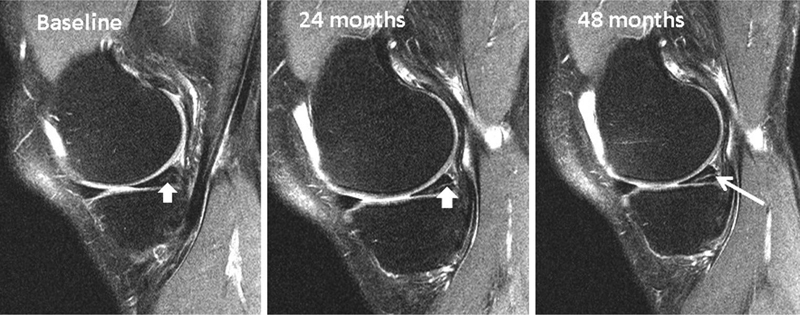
Development of an intrameniscus signal into a horizontal cleavage lesion in the posterior horn of a medial meniscus over a period of four years captured on repeat 3-Tesla knee MRI (courtesy of M Englund).
